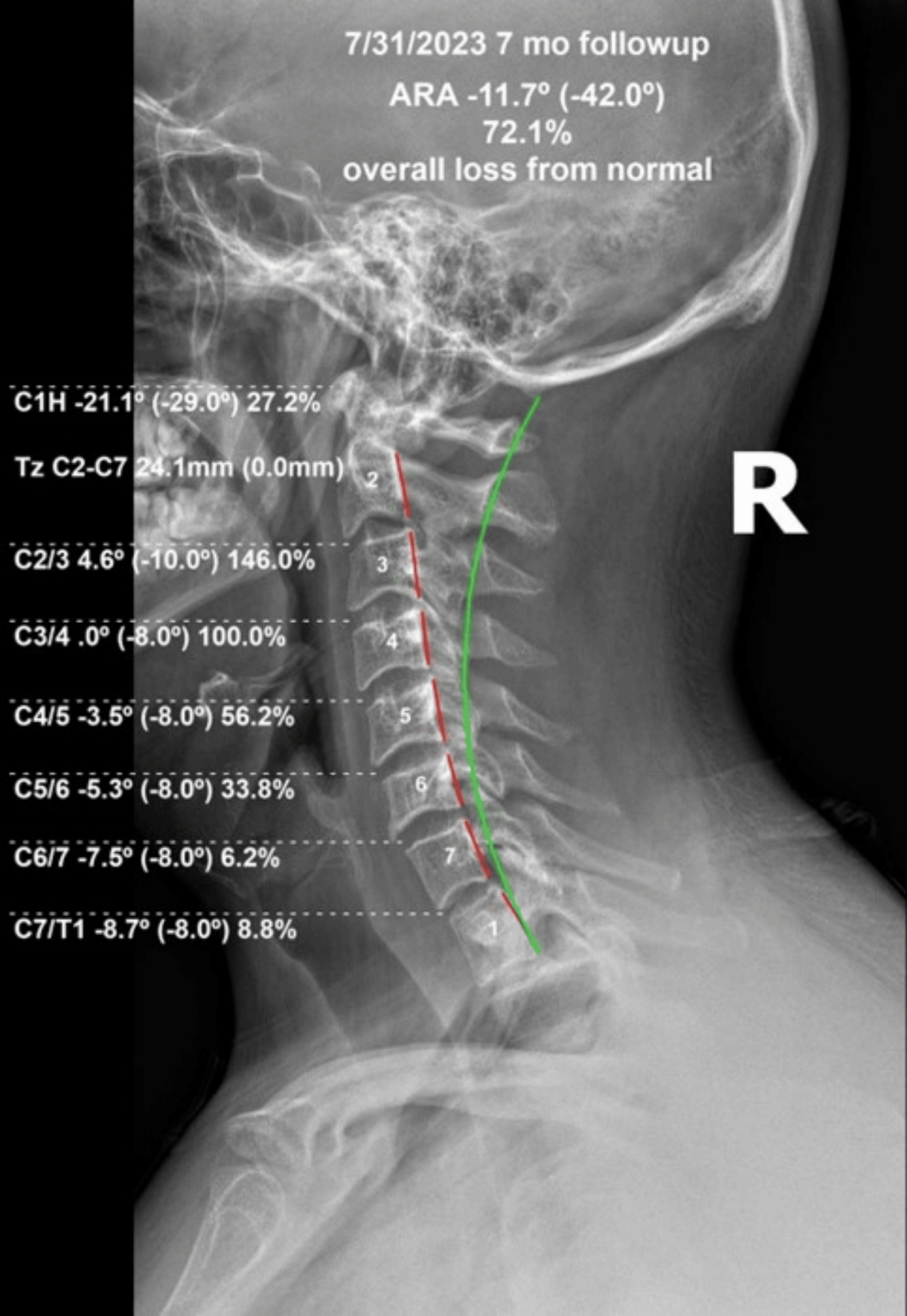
Follow-up re-examination lateral cervical radiograph after seven months of maintenance treatments. The cervical lordosis lost some of the initial correction and was -11.7° (vs. -1.3° initially), the atlas plane line was maintained at -21.1° (vs. -10.9° initially), and the anterior head translation maintained at 24.1 mm (vs. 35 mm initially). The kyphotic C2-C3 segment measured +4.6° (vs. +9.7° initially).
